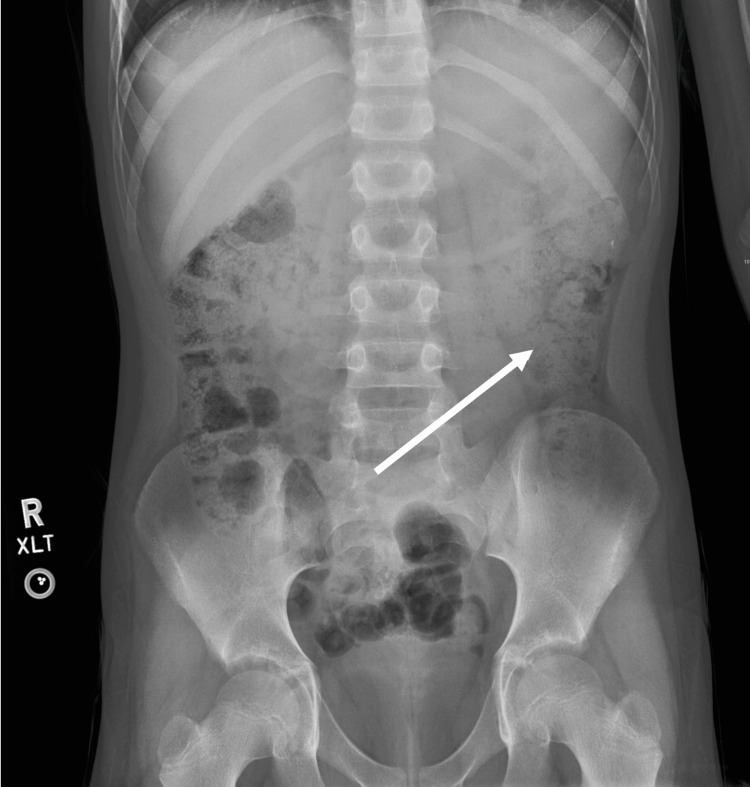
Abdominal KUB radiography demonstrating increased stool volume throughout the colon KUB: kidney-ureter-bladder The arrow points to increased stool burden in the descending colon.
